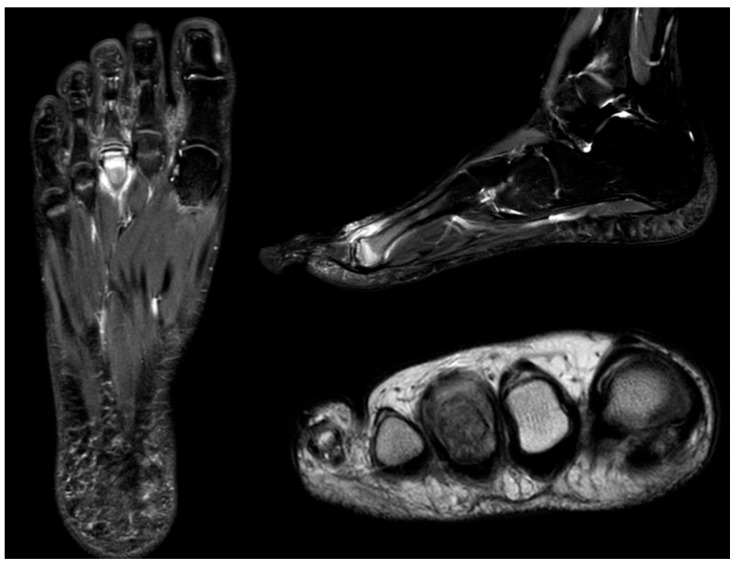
MRI of a 12-year-old female patient showing extensive bone marrow edema of the third metatarsal head, consistent with active inflammation and necrosis.
